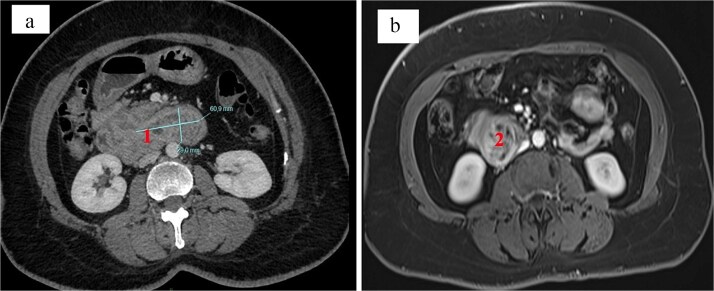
Appearance on imaging. Duodenal tumor on (a) CT scan and (b) MRI.
